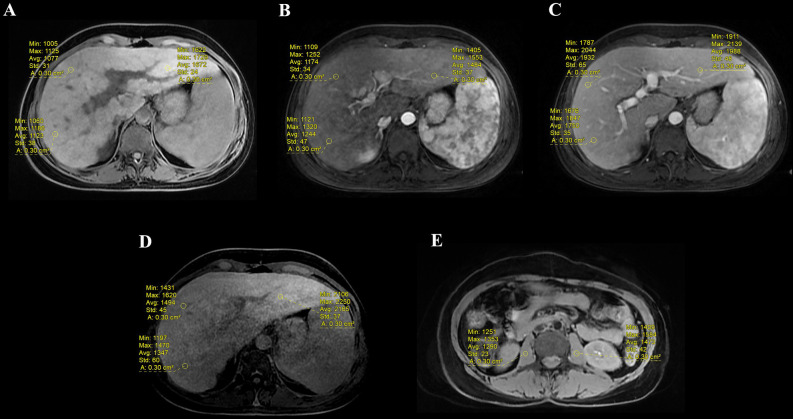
Signal intensity (SI) values in T1-weighted images before and after the injection of Gd-EOB-DTPA with operator-defined region-of-interest (ROI) drawn on the liver lobe. (A) SI on precontrast; (B) SI on portal venous phase (PVP); (C) SI on balance phase (EP); (D) SI on balance phase (HBP); (E) SI on paravertebral muscle (PM).
